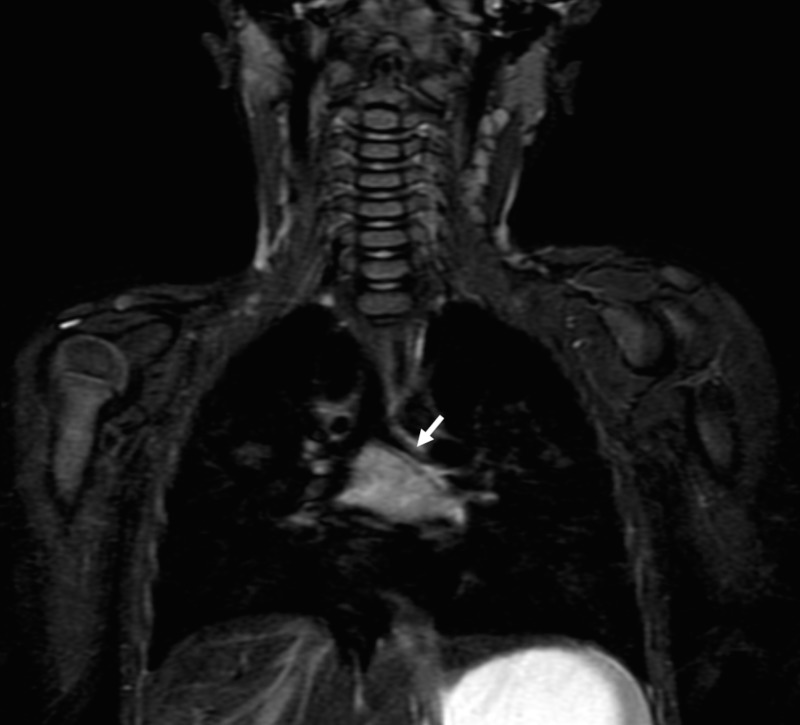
Coronal non-contrast T2 MRI image of mediastinal mass Coronal non-contrast T2 STIR (Short Tau inversion recovery) image shows a heterogeneous, mixed solid and cystic mass in subcarinal location causing elongation and at least moderate narrowing of the left main bronchus (arrow).
